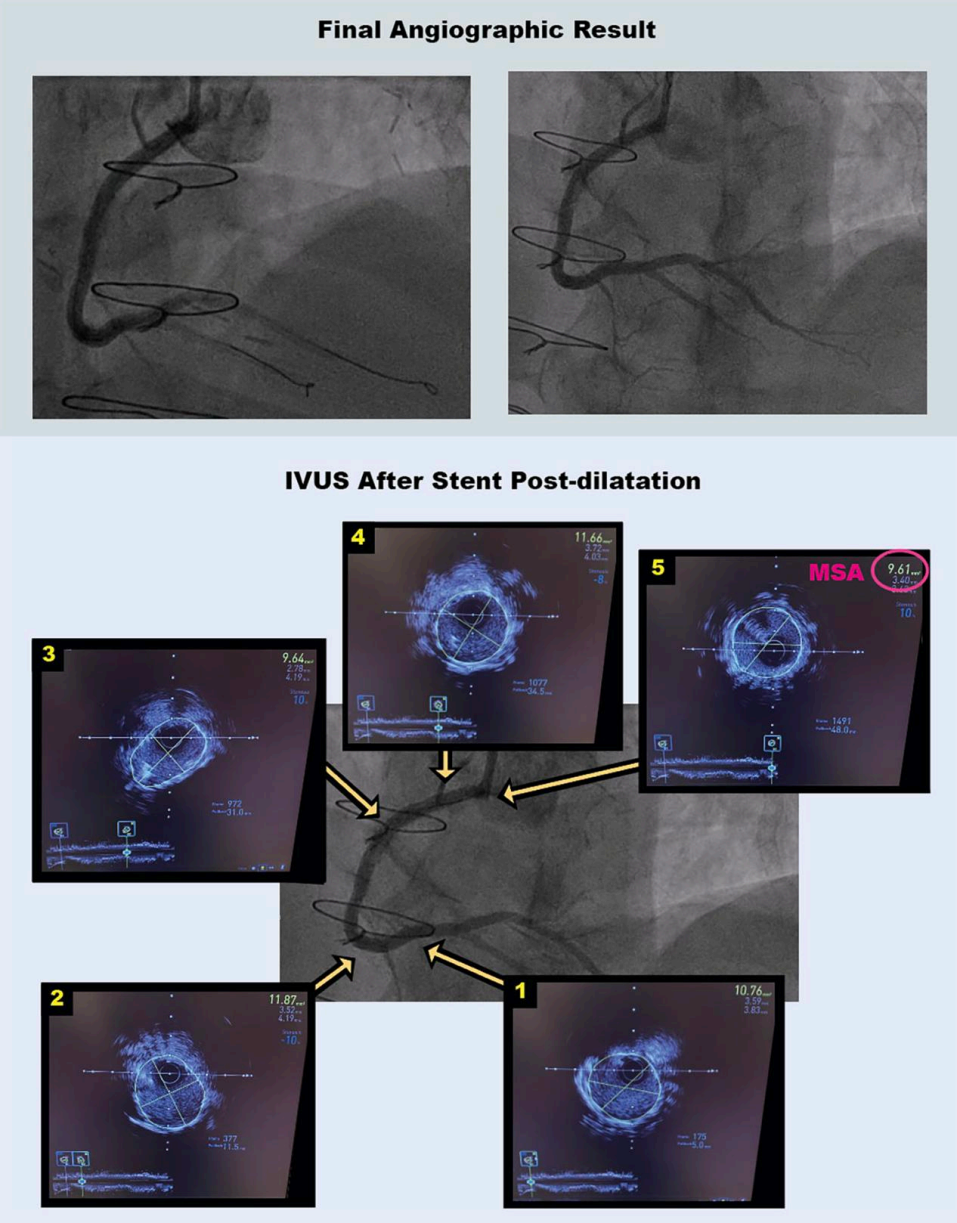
Final angiographic result and intravascular ultrasound post-percutaneous coronary intervention. IVUS, intravascular ultrasound; MSA, minimum stent area.
